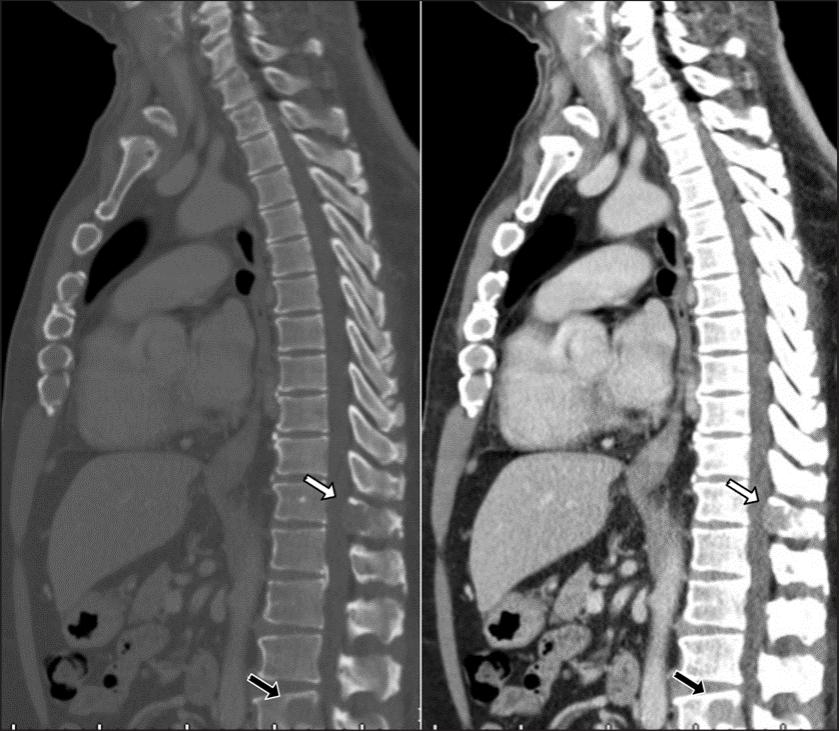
Sagittal CT images demonstrating osteolytic lesions with adjacent soft tissue component at the spinous process of T12 (white arrow) and posterior compartment of L4 (black arrow) vertebral body.
